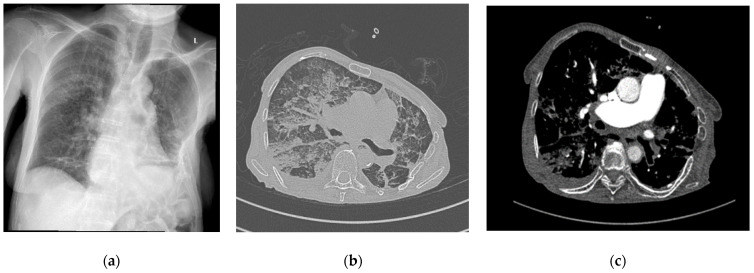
(a) Chest X-ray at admission; (b) contrast-enhanced chest CT scan lung window after surgery; (c) contrast-enhanced chest CT scan soft tissue window after surgery. L—left side.
